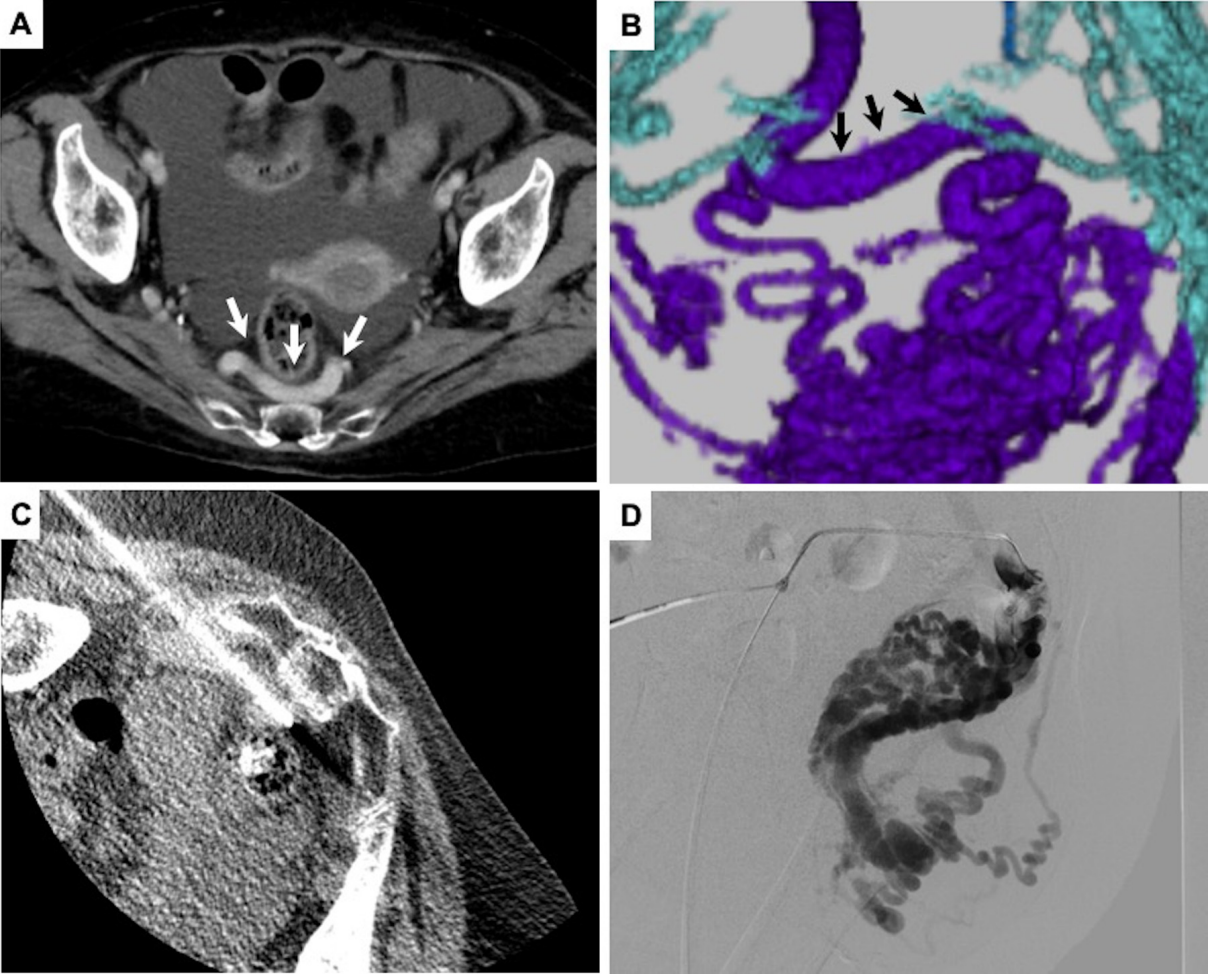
Contrast-enhanced computed tomography (CECT) revealed the dilatation of the right superior rectal vein (arrow) in (A) (axial view) and (B) (rear view of volume rendering). (C, A) direct puncture to the right superior rectal vein under CT fluoroscopic guidance. (D) Digital subtraction angiography from the right superior rectal vein showed large anorectal varices*.*
